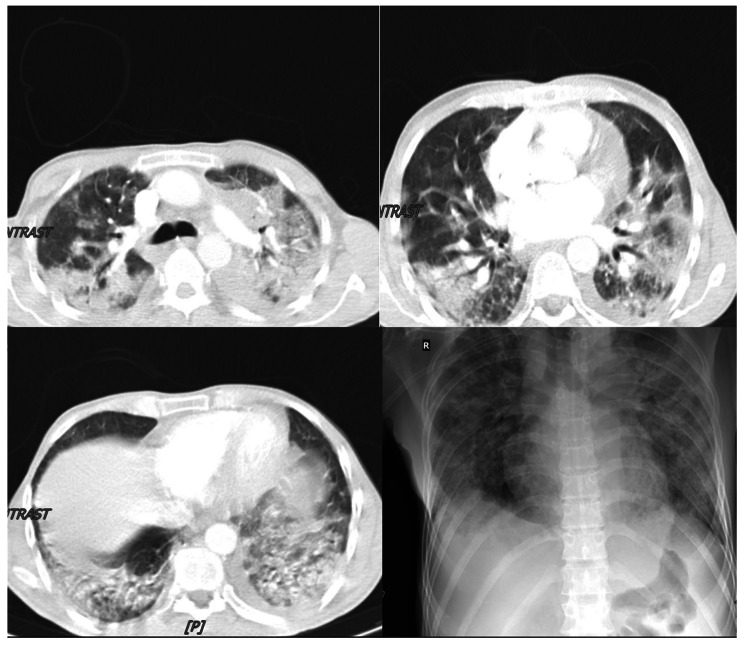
Chest CT scan of the patient on 06/10/2022
